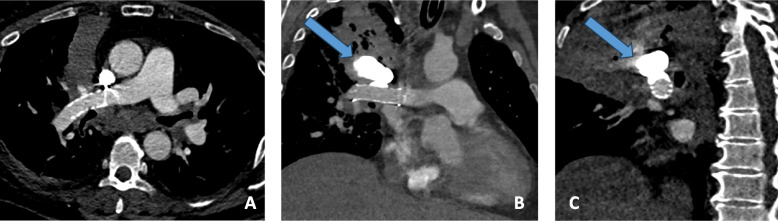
Follow-up CT scan showing a patent stent with a completely filled pseudoaneurysm using glue (blue arrow) in axial section A, coronal section B, and sagittal section C
